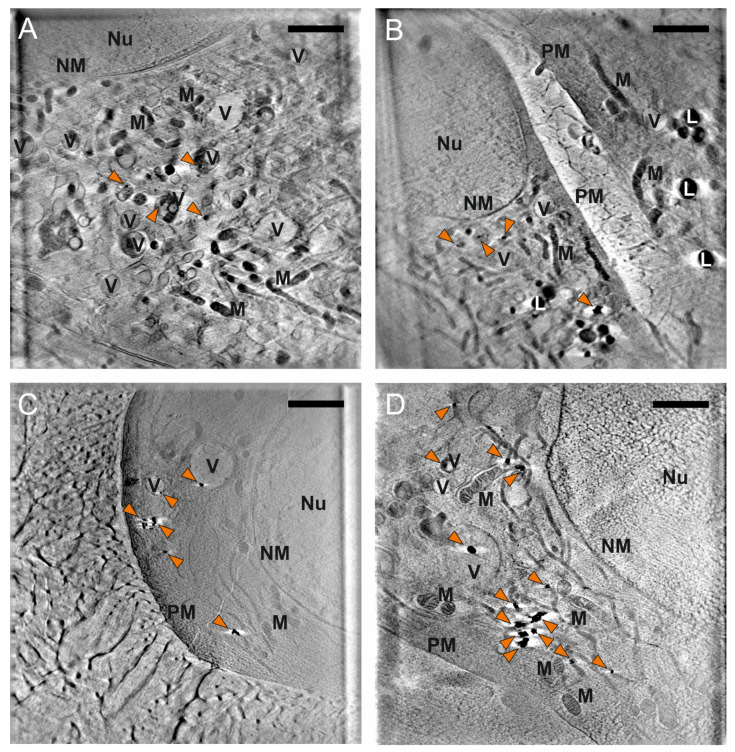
Slices of X-ray tomographic reconstruction of 3T3 (A,B) and J774 (C,D) cells incubated for 3 h with gold nanostars synthesized with 25 mM HEPES (NS25). The orange arrows indicate single particles or aggregates of nanostars. More examples are provided in Figure S3. Abbreviations: Nu, nucleus; NM, nuclear membrane; V, vesicles; M, mitochondria; L, lipid droplets; PM, plasma membrane. Scale bars: 2 µm.
